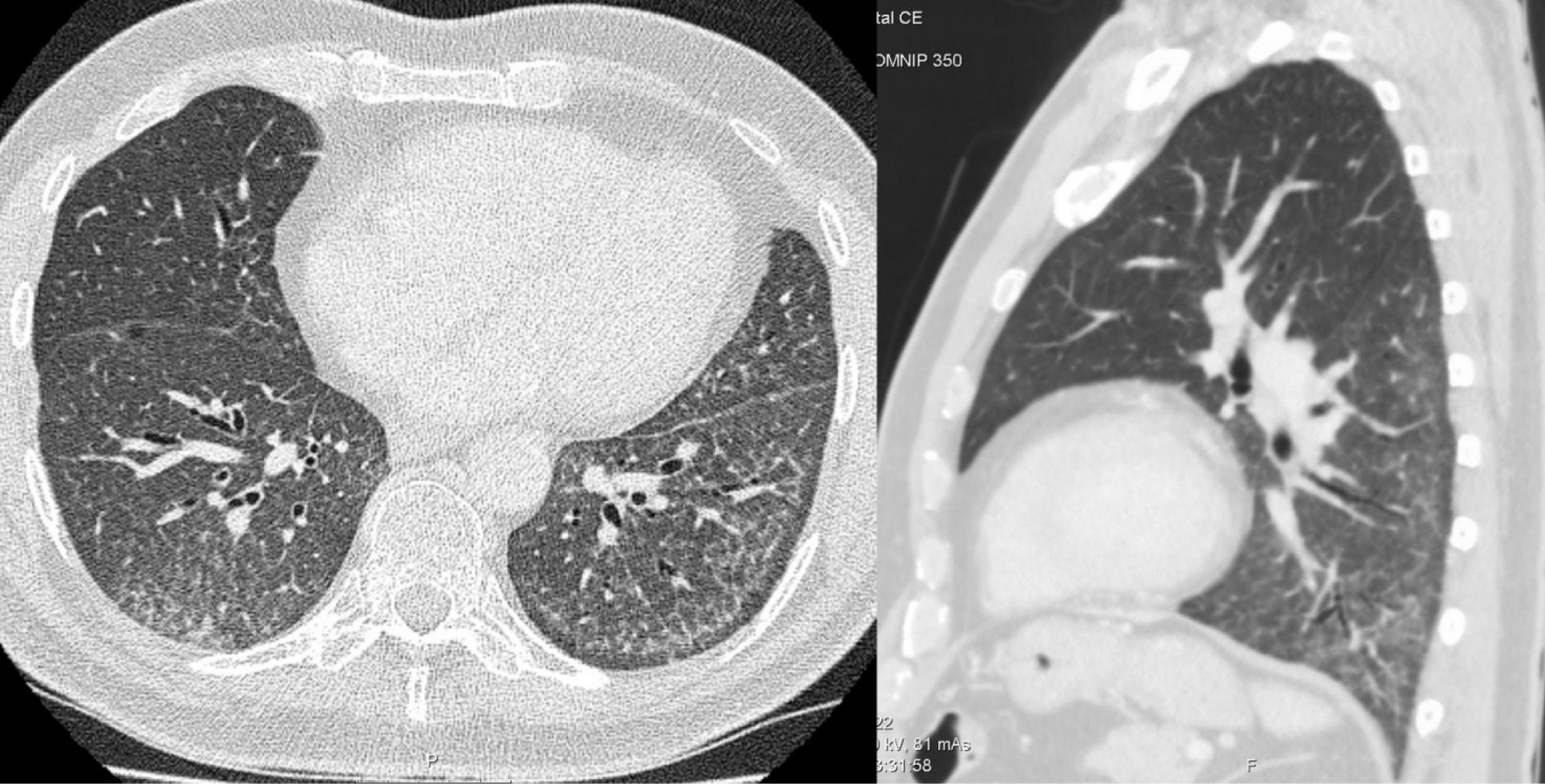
HRCT of the lungs showing diffuse ground glass opacity and fine reticulation with sparing of the immediate subpleural lung tissue on axial and sagittal planes.
